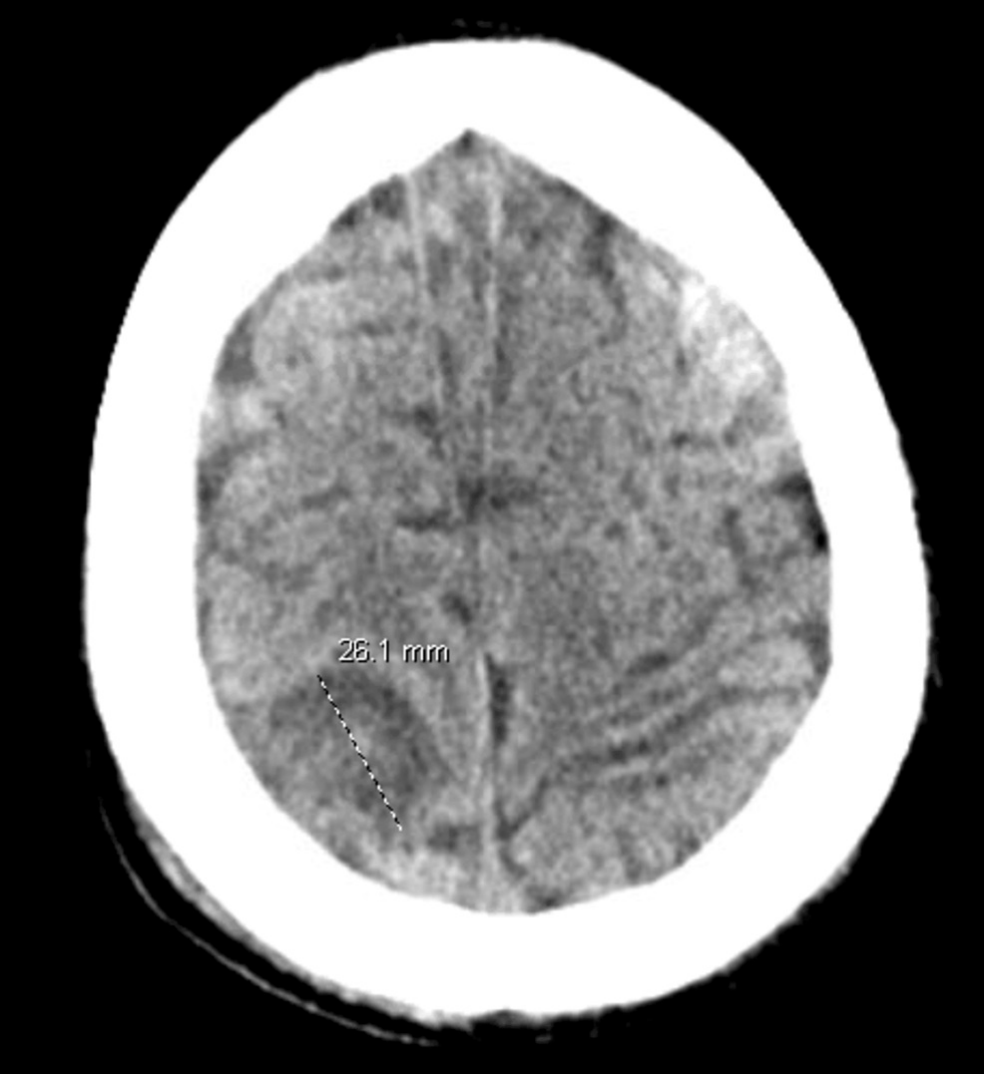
Axial view of non-contrast CT head with 2.6cm rounded area of hypoattenuation with mild mass effect in the posterior right frontal lobe
